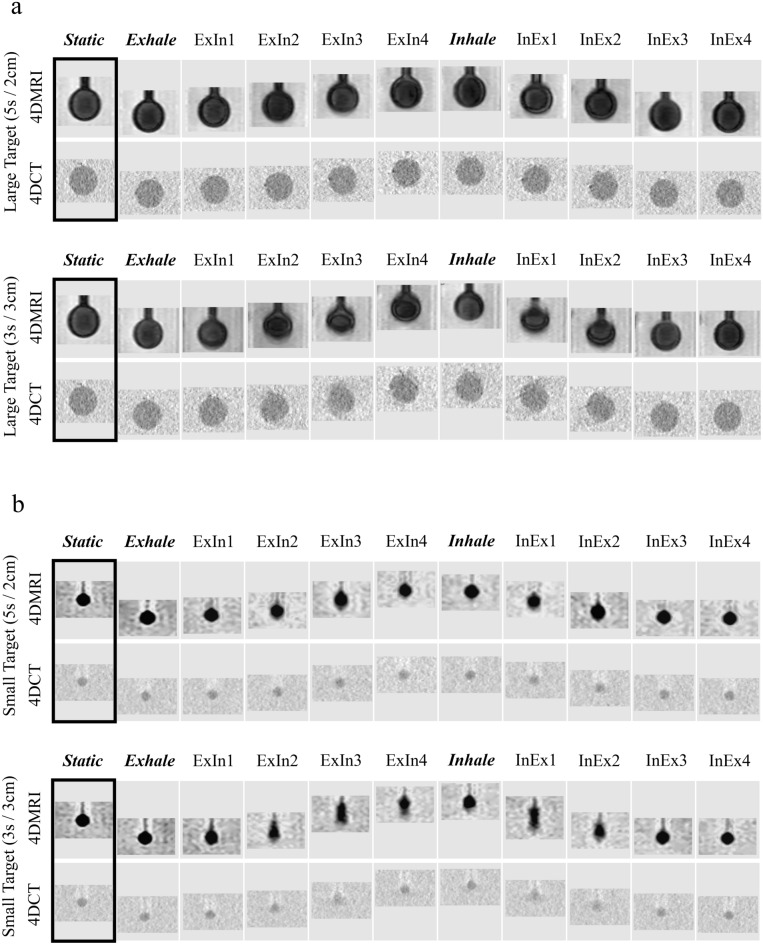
Acquired 4D-MRI and 4D-CT images with ten respiratory phases in a regular motion pattern (5 s/2 cm) and fast motion pattern (3 s/3 cm). The upper and lower panels show the results of large and small targets, respectively. The static image as a reference is shown on the left. ExIn refers to the phase from exhaling to inhaling, and InEx refers to the phase from inhaling to exhaling.
